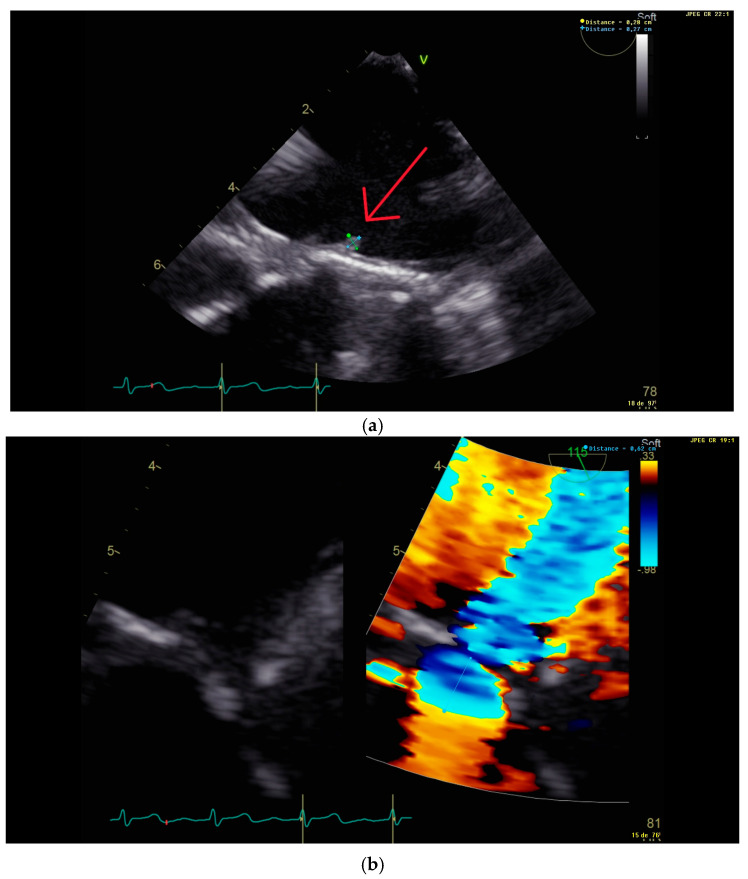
Trans-esophageal echocardiography performed 2 months after the second valvular replacement, showing (a) a 3 mm vegetation (red arrow) on the new prosthetic mitral valve (below) and (b) mitral insufficiency with a proximal isovelocity surface area of 6 mm.
